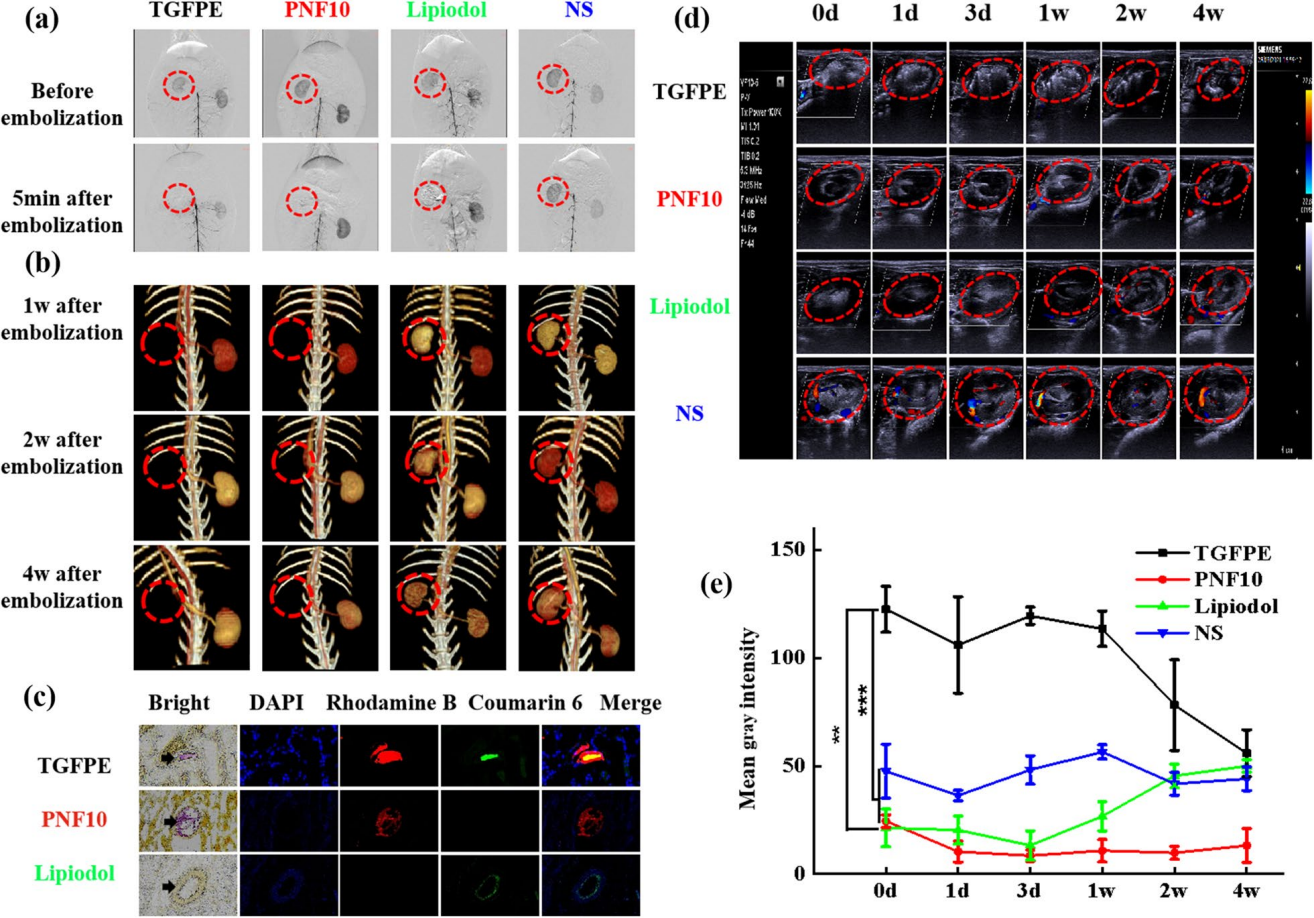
Renal artery embolization and ultrasound imaging comparison of TGFPE, PNF10 dispersion, lipiodol and NS. a DSA images of kidney at different points of time after vascular embolization with the TGFPE, PNF10 dispersion, lipiodol and NS. b 3D-CT images of kidney at different points of time after vascular embolization with the TGFPE, PNF10 dispersion, lipiodol and NS. c Bright field and fluorescence scanning of isolated embolized kidney slices within 1 d after vascular embolization (arrow pointing to blood vessel). d Color doppler and B-mode ultrasound images of kidney at different points of time after being embolized by the above embolic material or the injection of NS. e The statistical analysis of B-mode ultrasound images in kidneys at different points of time after being embolized by the above embolic material or the injection of NS. The region surrounded by dotted red line is the embolized kidney. *P* < 0.01, P* < 0.001
